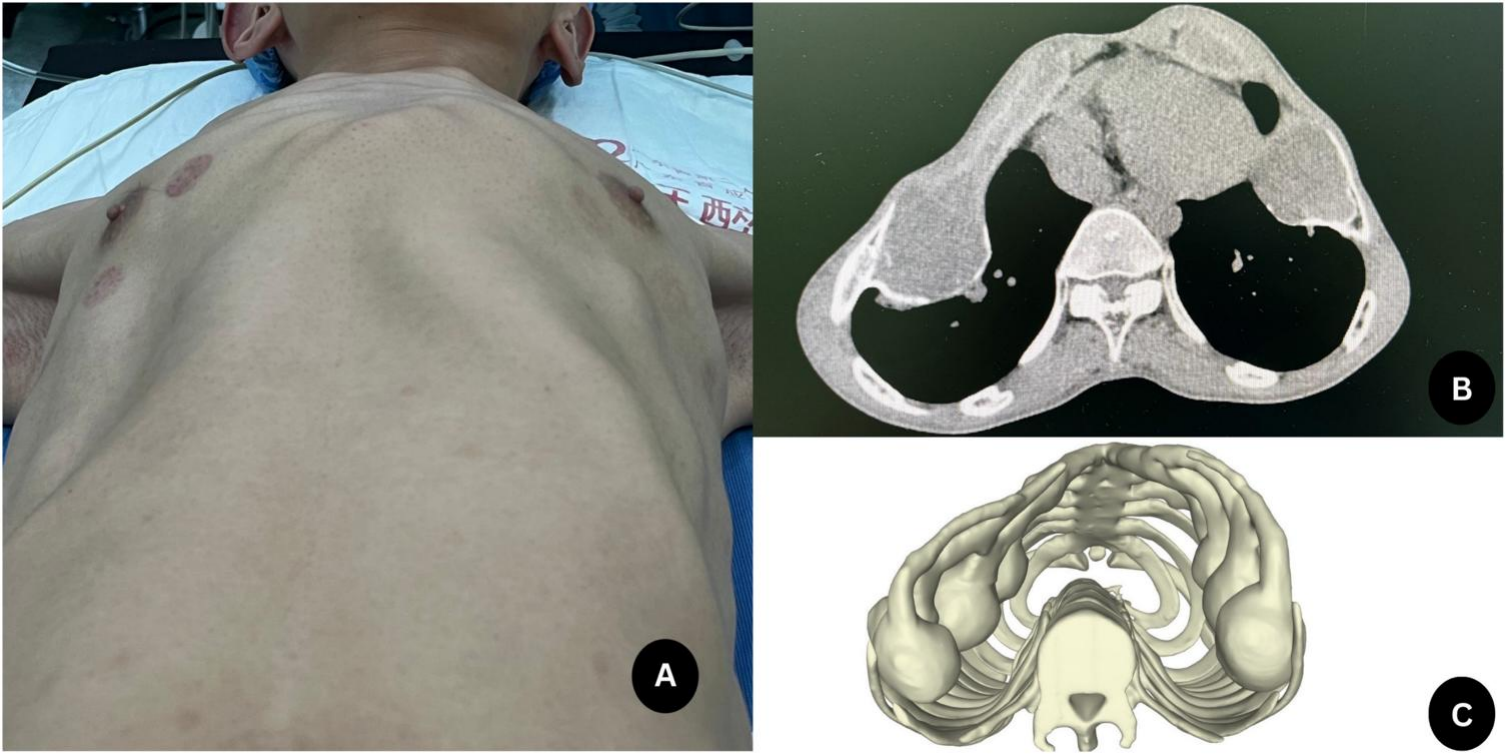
Appearance of the Chest Wall and Computed Tomography Images of the Patient. Preoperative chest wall appearance (A); preoperative chest computed tomography image (B); and 3-dimensional reconstruction image (C).
